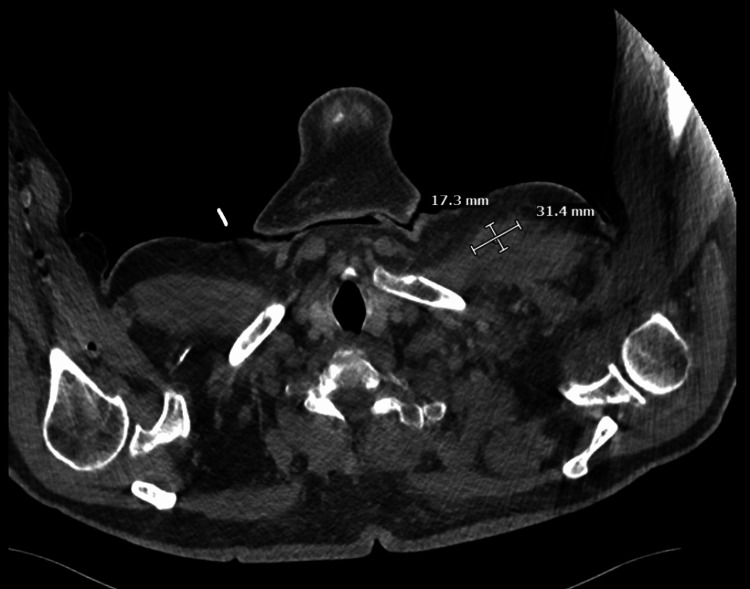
Computed tomography (CT) axial plane imaging of the upper chest demonstrating mild skin thickening and subdermal stranding, with a 1.7 x 3.1 x 2.7 cm underlying fluid collection.
